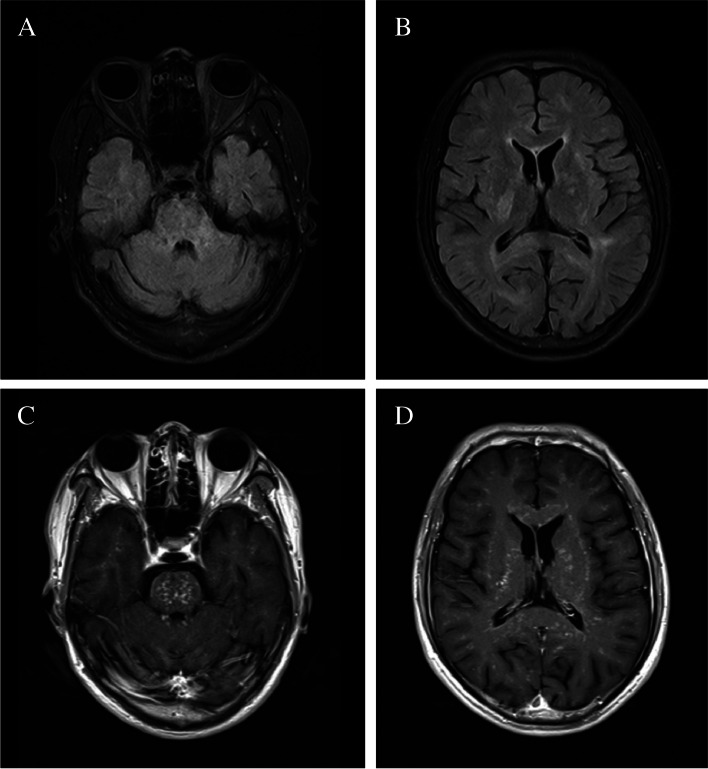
Coronal T2-weighted brain MRI demonstrates multiple lesions in the pons, basal ganglia and cerebral hemisphere with relative hyperintensity (A and B), which showed uniform enhancement with gadolinium contrast agent (C and D)
